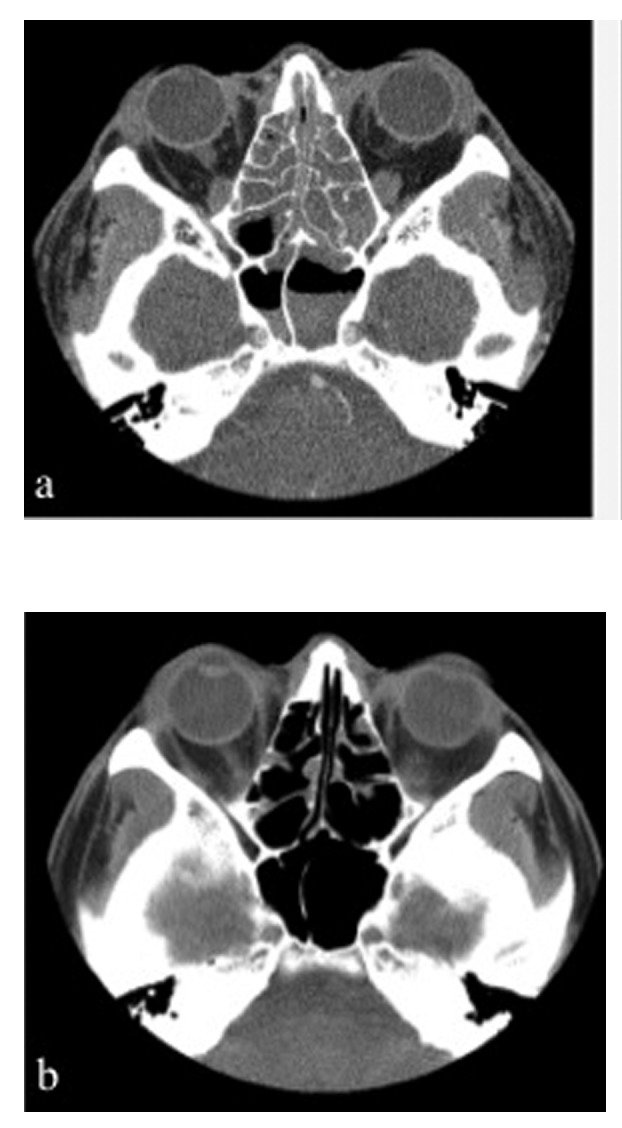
A 29-year-old male received a total dose of 50 Gy and 6 cycles of dexamethasone, etoposide, ifosfamide and carboplatin (DeVIC). (a) Computed tomography (CT) scan prior to chemoradiotherapy (CRT) shows a naso-paranasal space-occupying soft tissue mass. (b) The soft tissue mass completely disappeared 1 month after the completion of CRT.
